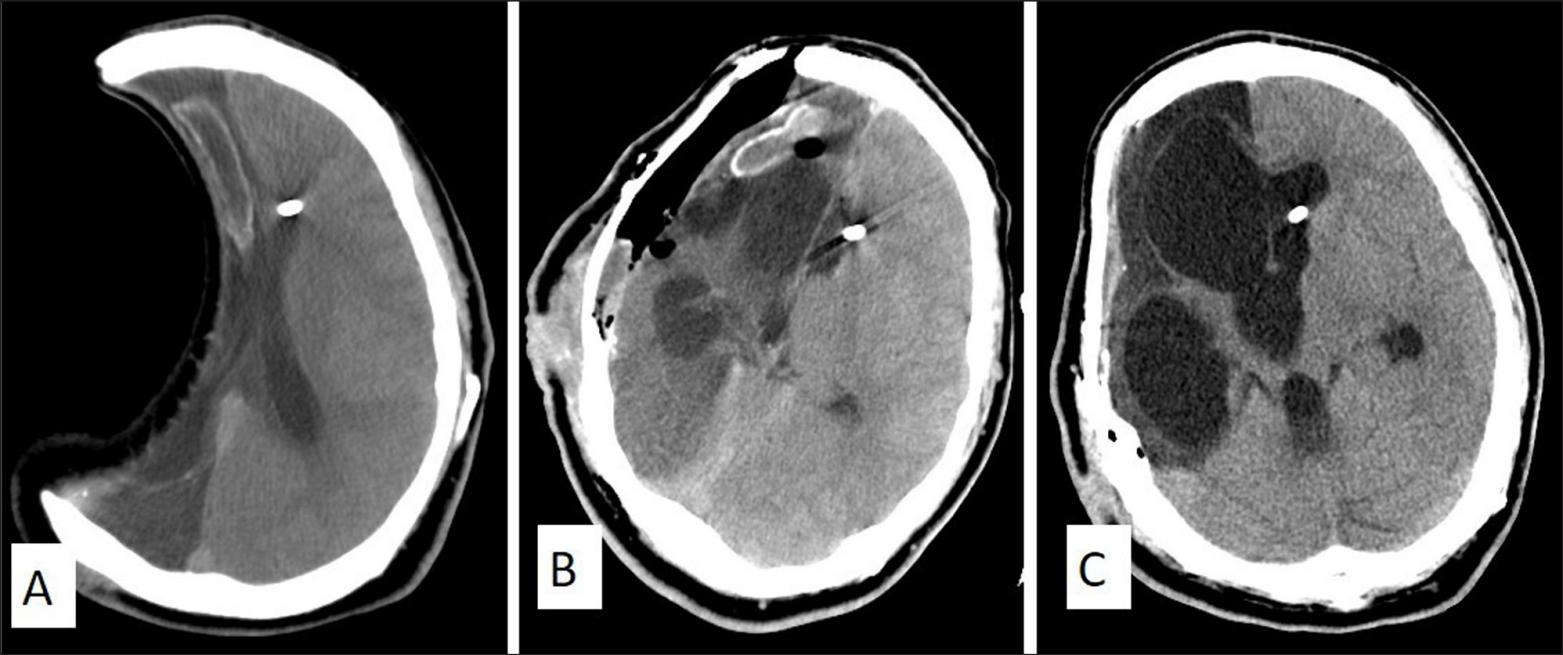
Axial brain CT of a 22-year-old man who underwent right hemicraniectomy. After resolution of the cerebral edema and mass effect, the pathological side of the brain appears “sunken” (A). This appearance is also known as “Motor Trephine Syndrome (MTS)” or “Sunken Brain and Scalp Flap Syndrome” (SSFS). This condition is further aggravated by CSF diversion procedures such as ventriculo-peritoneal shunt. Note of the ventricular catheter tip in the left frontal horn. He subsequently underwent titanium cranioplasty with pneumocephalus underneath the titanium implant (B). There is interval improvement following cranioplasty with improvement of the patients’ neurological and cognitive functions. A few weeks later, he developed a late postoperative complication with hydrocephalus due to shunt dysfunction (C).
